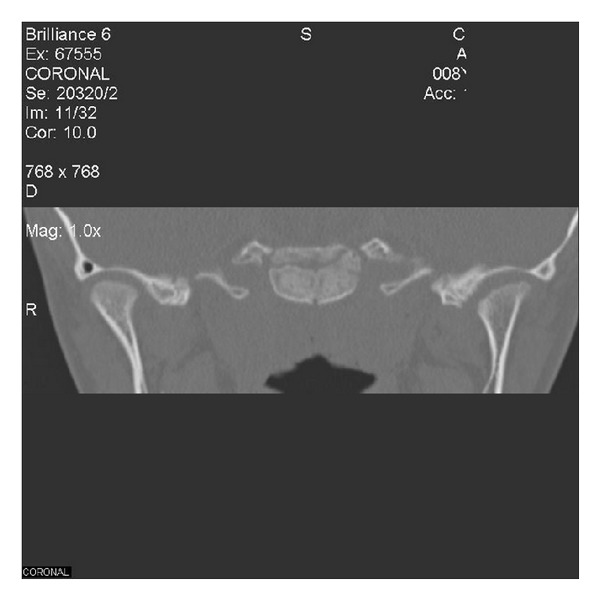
Coronal sectional CT showing flattening of the left condyle.
